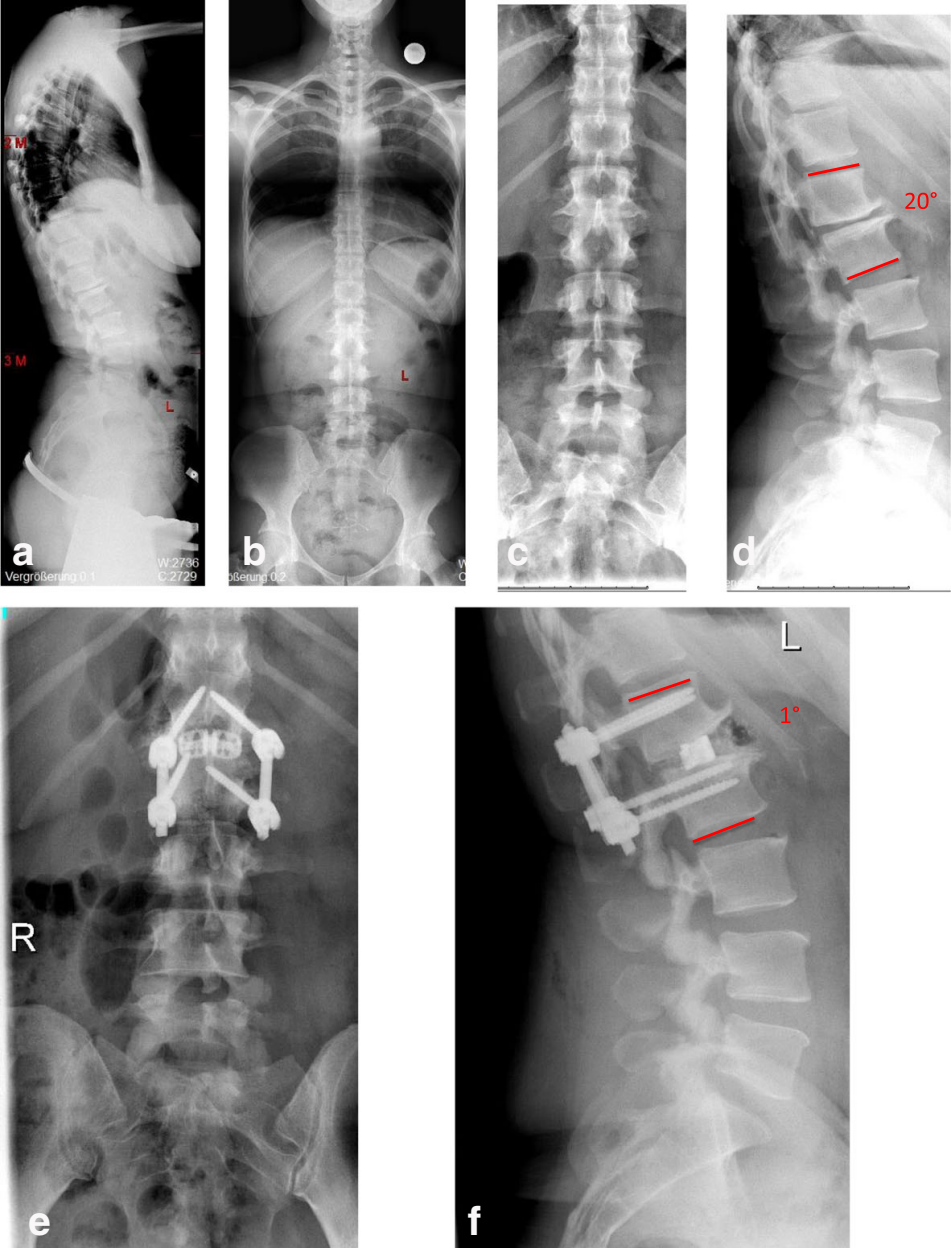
42- year old female with status post L1 fracture and segmental kyphosis L1/2. Figures a/b display the whole spine x-rays, Figures c/d the lumbar x-rays in both planes including the measurements of the deformity. Figures e/f show the postop standing x-rays of the lumbar spine including the measurements in both planes
